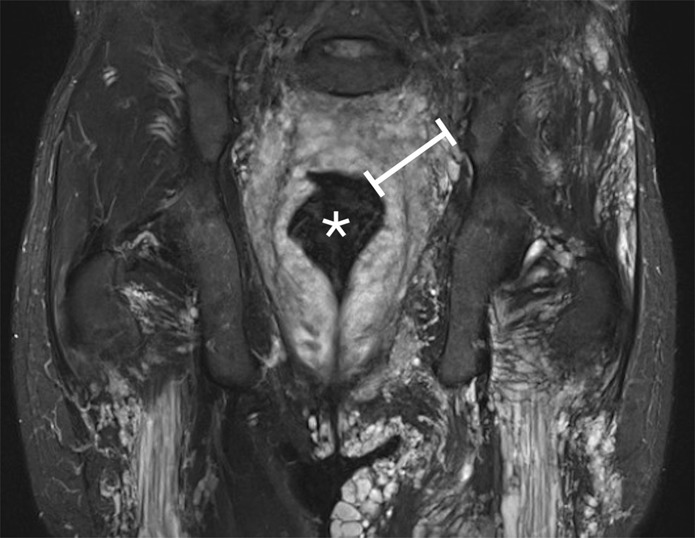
MRI of a 35-year old patient with expanded VM, both rectosigmoidal and in lower limbs. (*) Rectosigmoidal lumen; (I) rectosigmoidal intramural VMs associated with massively dilated rectosigmoidal wall.
